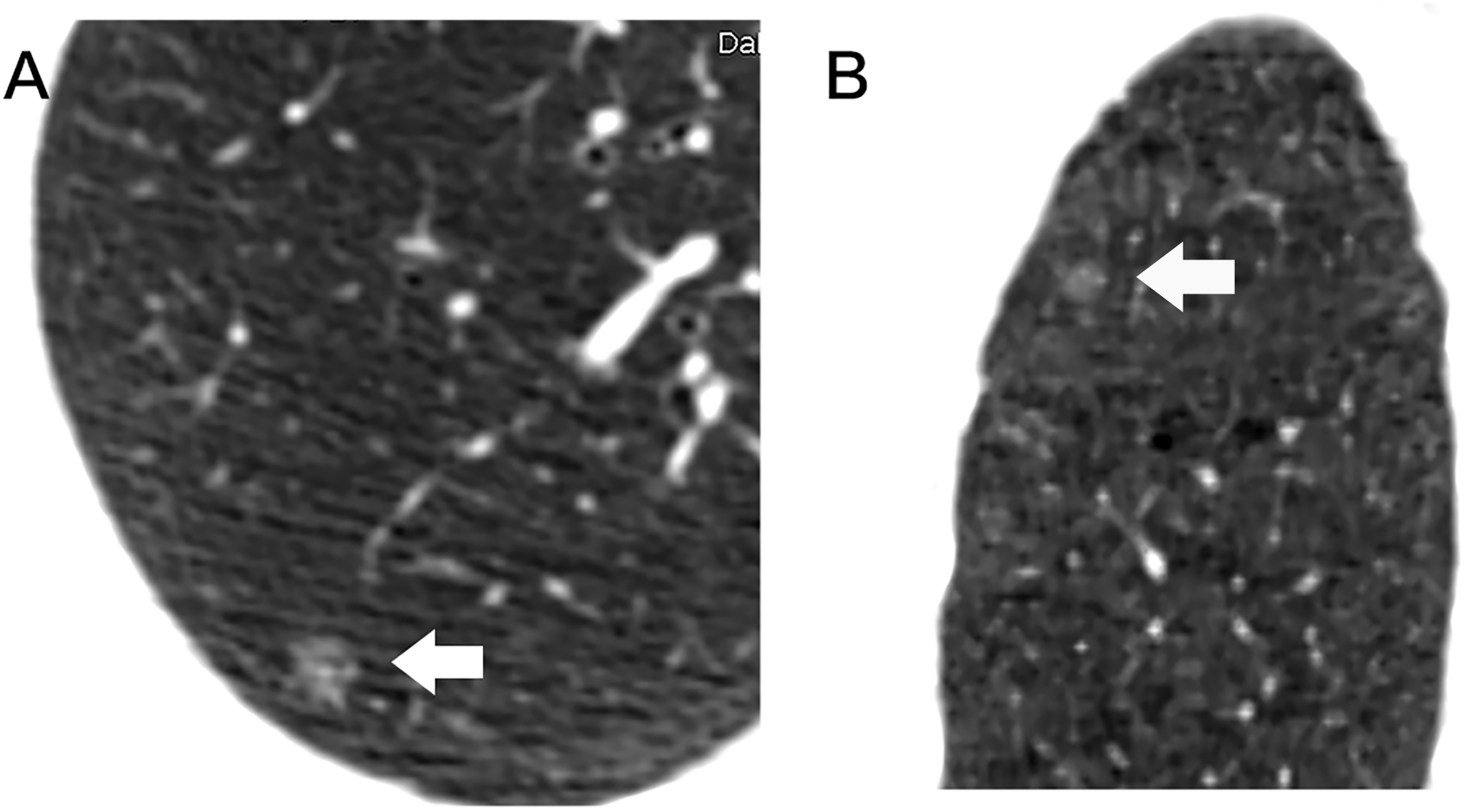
Radiologic findings of pure GGN. A 58-year-old woman with an AAH in the upper lobe of right lung. Axial (A) and coronal (B) HRCT images show a pure GGN with a round shape and a smooth border, and a vessel through pure GGN (type I) (coarse arrow).
